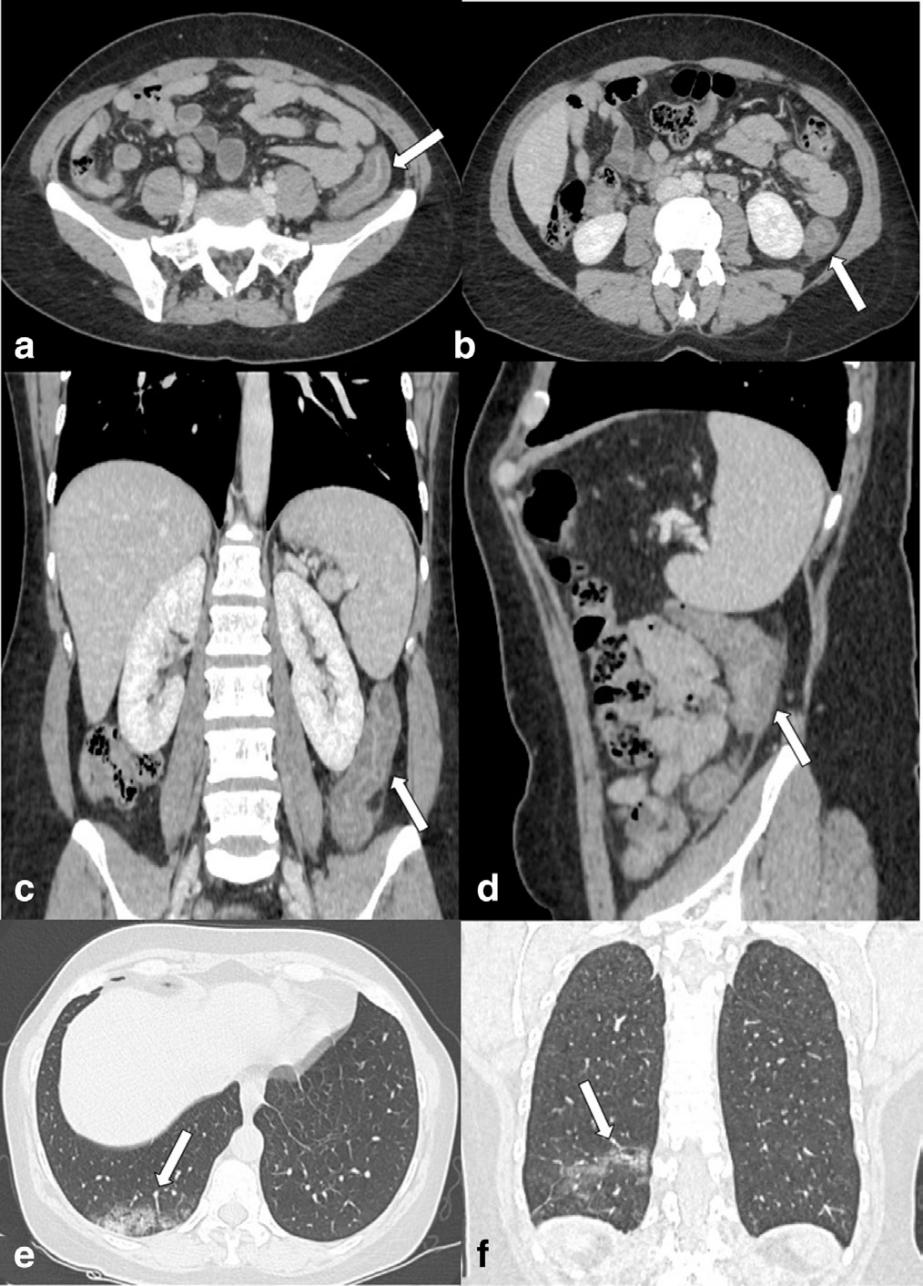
Enhanced CT scan (portal phase) of the abdomen of a 55-yo female admitted to
